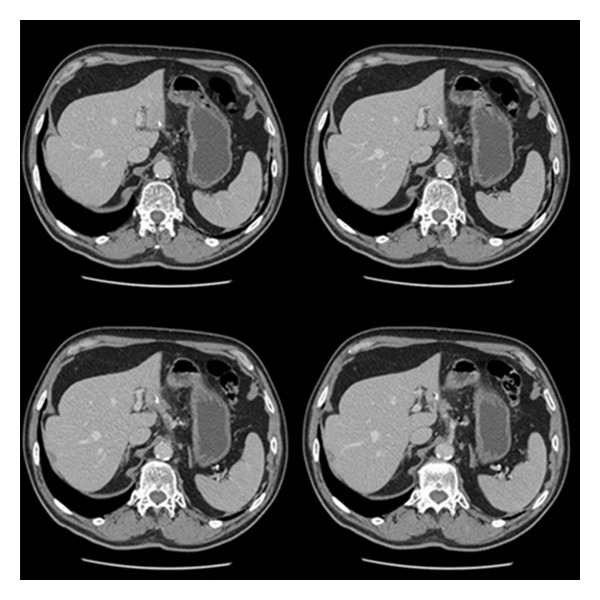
CT scan: presence of a linear radiopaque structure 3 cm in length at the inferior margin of left lobe of the liver.
